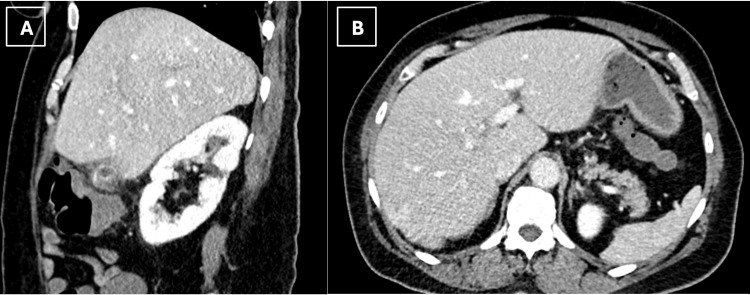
Sagittal (A) and axial (B) CT abdomen and pelvis showing recurrent cholecystitis after subtotal cholecystectomy and interval resolution of previous hepatic abscesses
